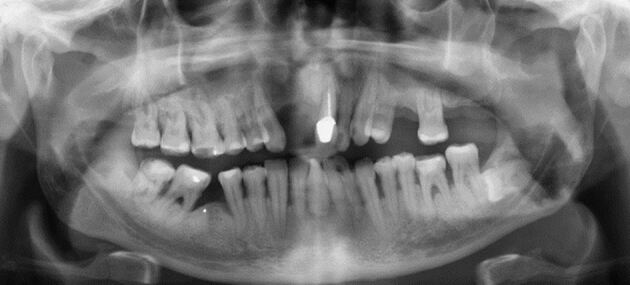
Orthopantomogram of the patient’s dentition
